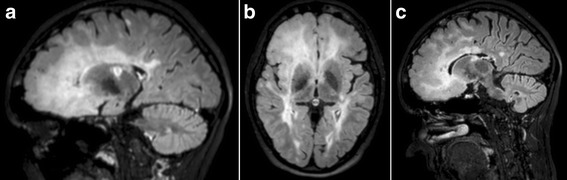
a FLAIR, Fluid-Attenuated Inversion Recovery, sagittal (2014): Large non-homogenous hyperintense lesion of the right frontal lobe involving demyelination and oedema with mild mass-effect. It is relatively sharply defined to grey matter and confluent with white matter. Glial tumour is undetectable. b FLAIR, Fluid -Attenuated Inversion Recovery, transverse (2014): Diffuse hyperintense lesion of the right frontal lobe - demyelination. It has mild mass-effect. It is well-defined to cortex and to white matter and irregular in shape. c 3D DIR, 3D Double Inversion Recovery, sagittal (2014): Multifocal cortical involvement in the right frontal cortex adjacent to demyelinated lesions, diffuse confluent hyperintensive lesion in cortico-subcortical fronto-polar region
